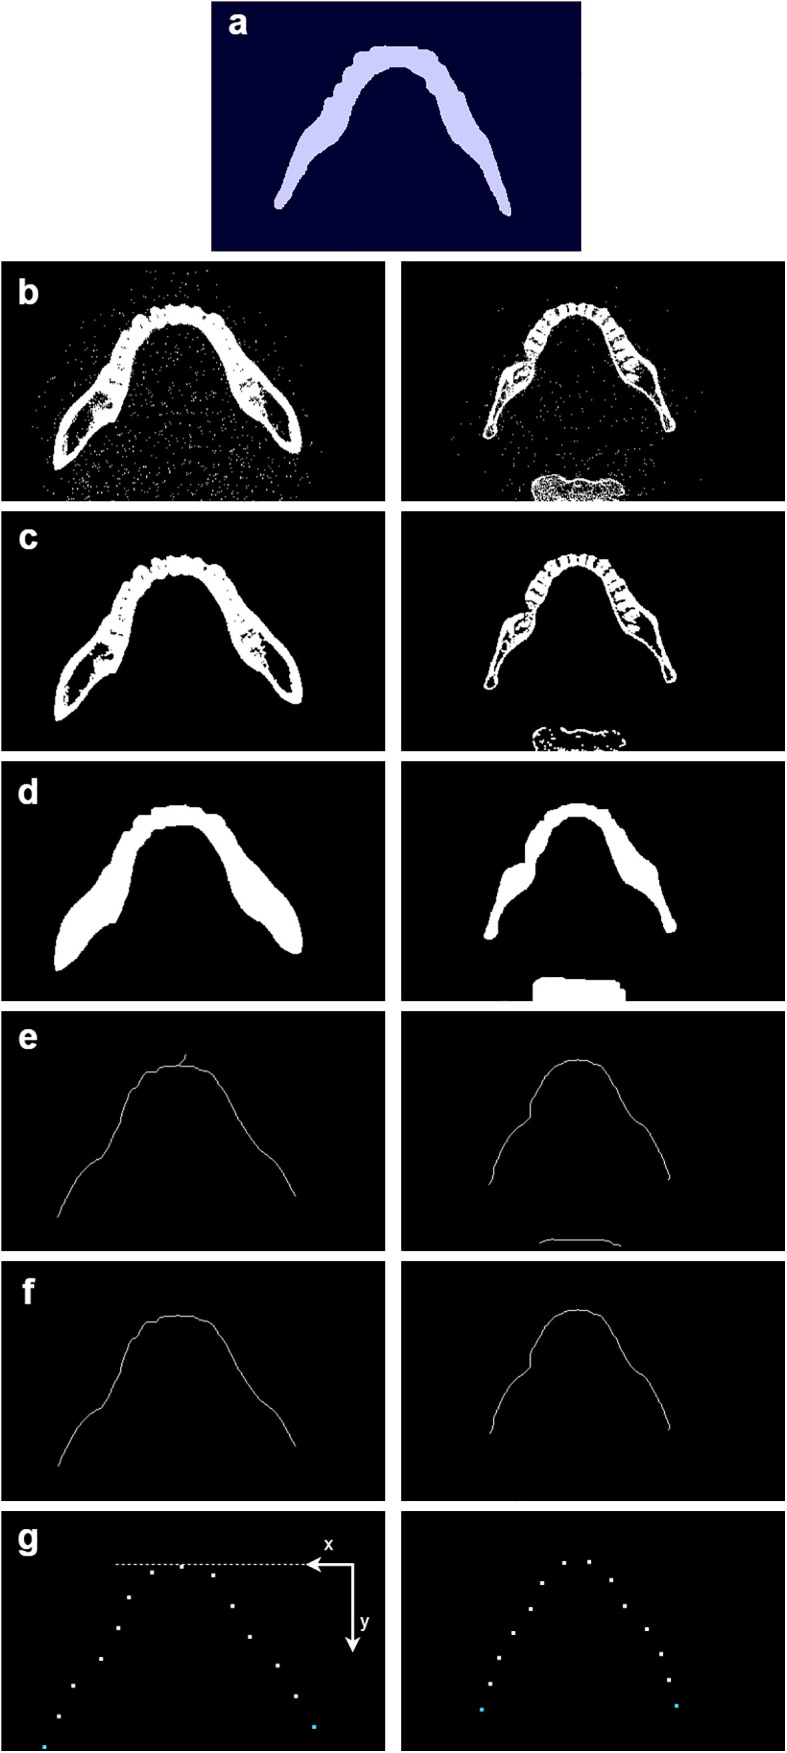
Steps in the extraction of 14 coordinates from the CT slices for the curved MPR. It shows the step-by-step transformation for two examples on the left and right sides: a) a binary mask of the lower dental arch is shown, which is compared with the binarized CT images to obtain the best slice containing the lower jaw, as shown in b). The image is then cleaned in c) using morphological filtering with an *opening* operation to remove small-scale noise. Subsequent to this, morphological filtering with a *closing* operation is applied, where gaps or inconsistencies in the outline are filled to improve the overall shape of the jaw, as shown in d). In e), the image is skeletonized by creating a line along the center of the lower jaw. Protruding paths are then eliminated using graph-based optimization algorithms, as demonstrated in f). Along this optimized curve, 12 evenly spaced coordinates are extracted, and the curve is extended with an additional coordinate at each end, represented by the blue points in g).
